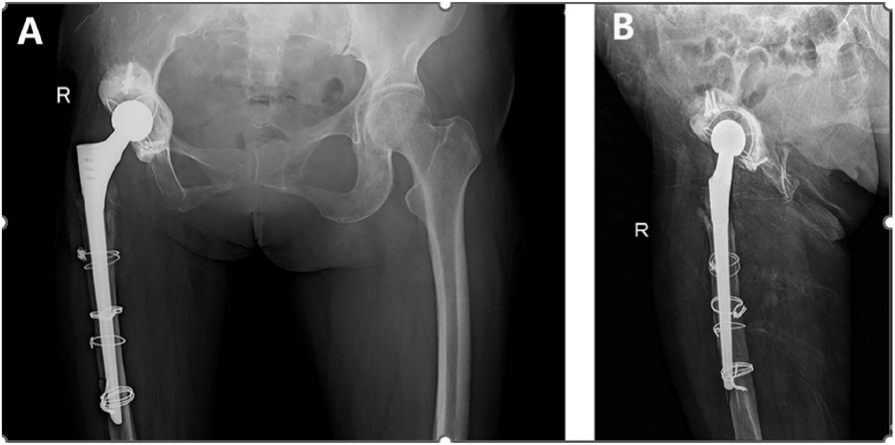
A Anteroposterior X-ray: due to excessive acetabular bone defects, the acetabulum is fixed using the bone cement technique. B Lateral X-ray: a long-stemmed cemented stem is implanted on the femoral side, with a stress fracture in the distal part of the stem
